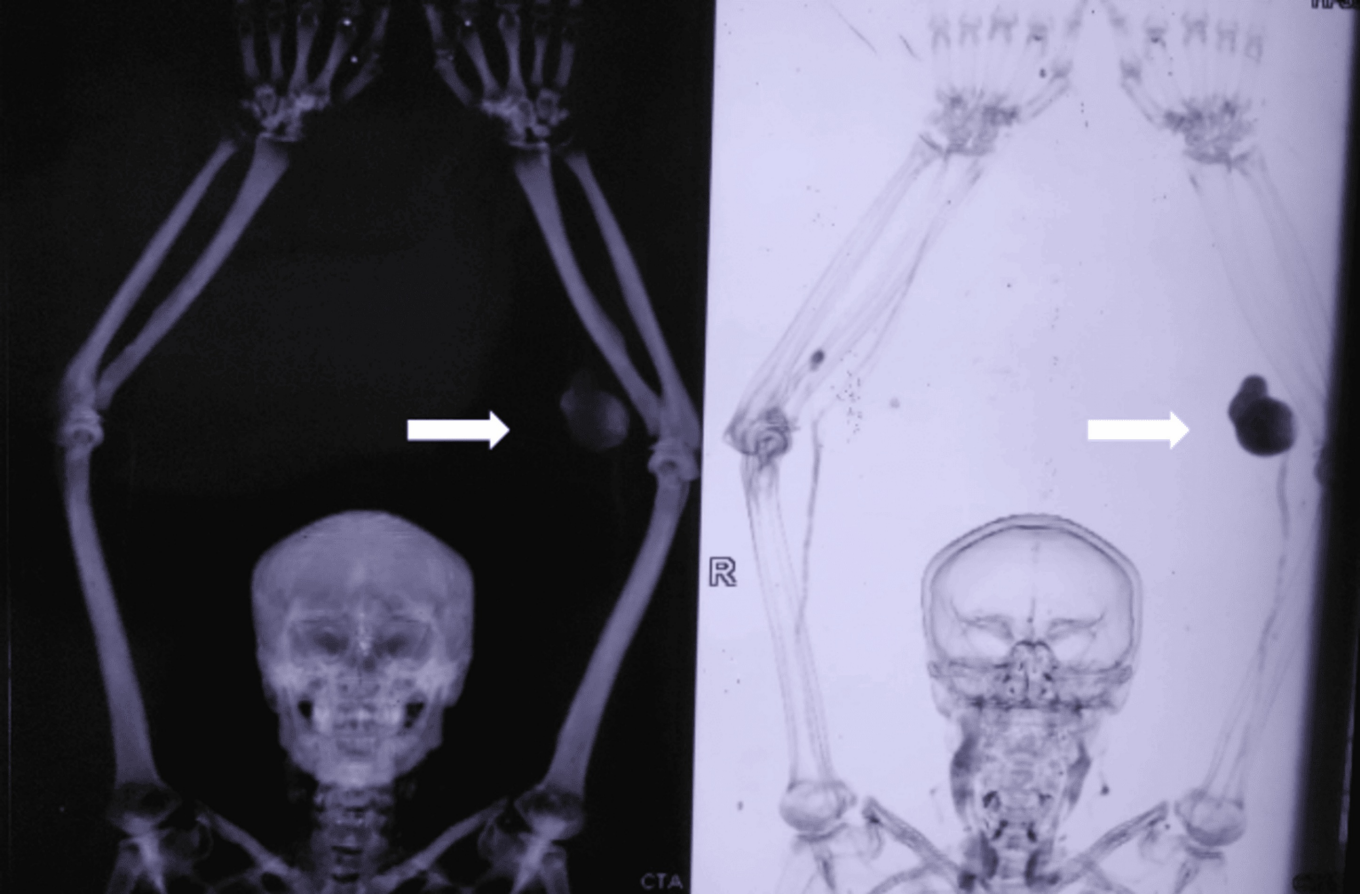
CT angiogram highlighting the B/L upper limb peripheral artery aneurysm
